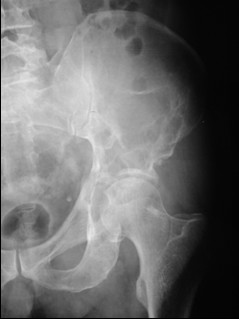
Preoperative plain radiographs showing wide acetabular osteolysis.
